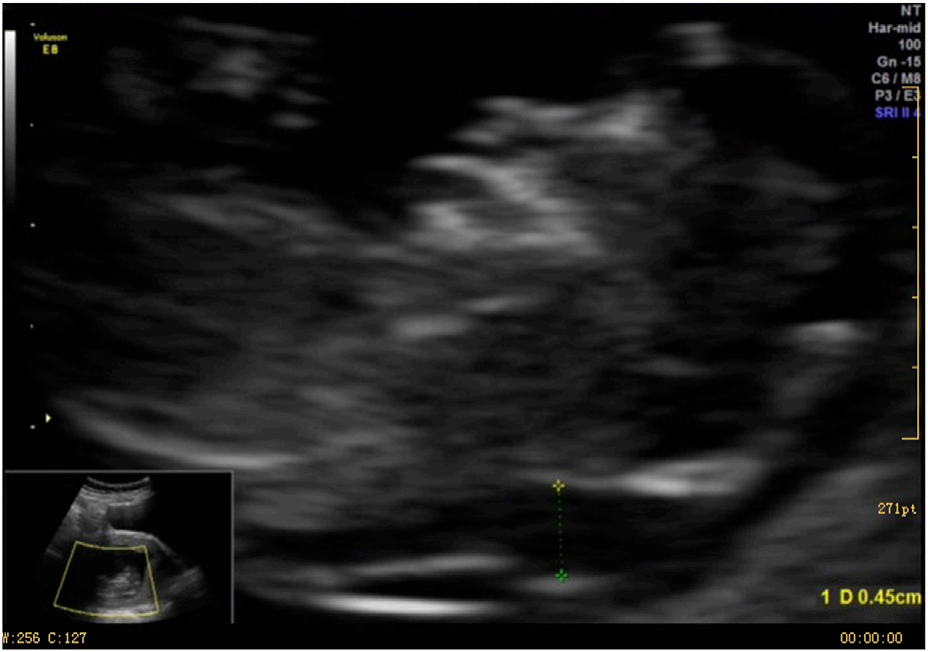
2D sonographic image of the fetus at 12^+2^ weeks showing NT = 4.5 mm (vertical section).
